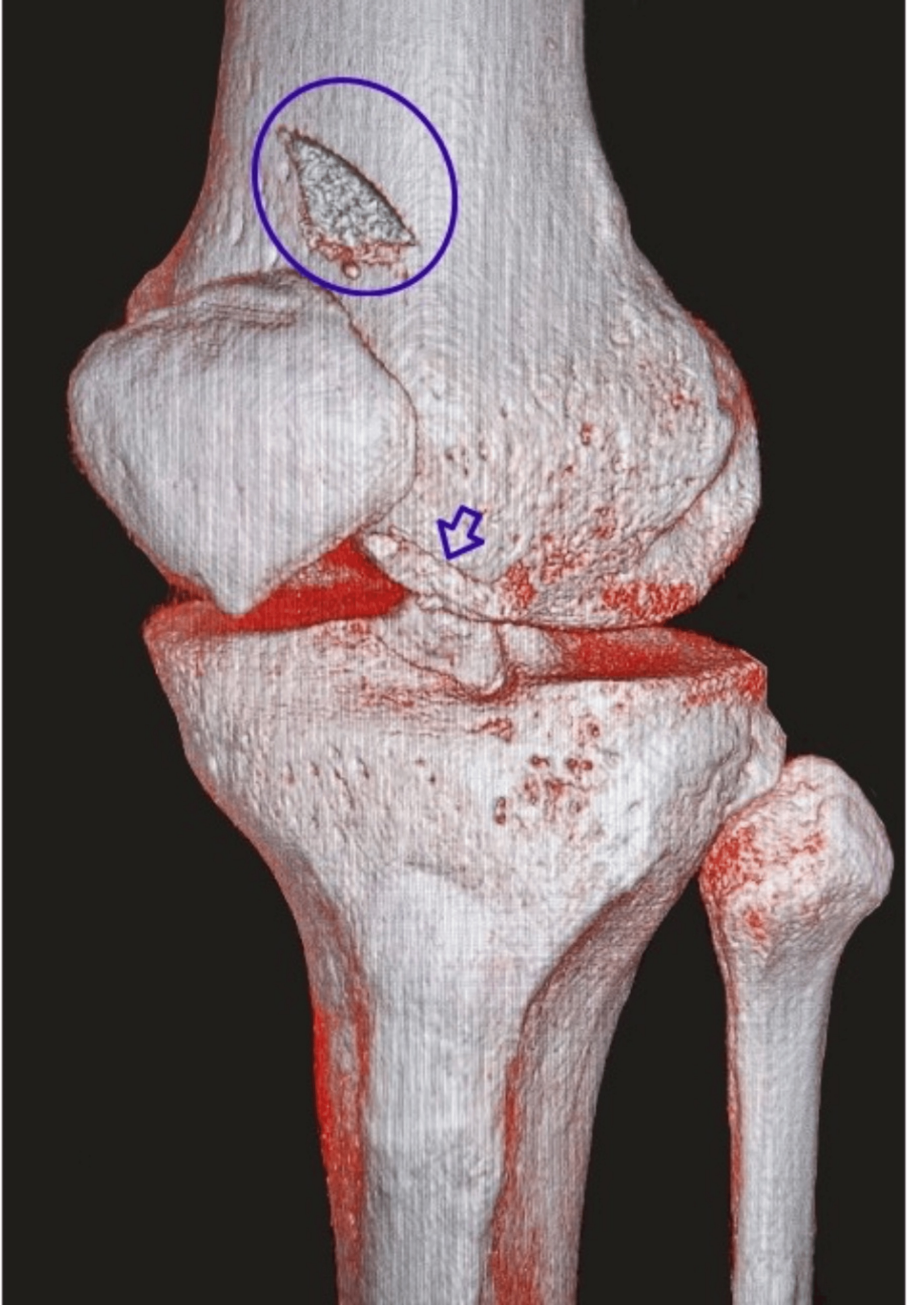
3D reconstruction from the CT scan performed at initial presentation, with the blue circle highlighting the osteochondral fragment and the blue arrow indicating the trochlear defect.
